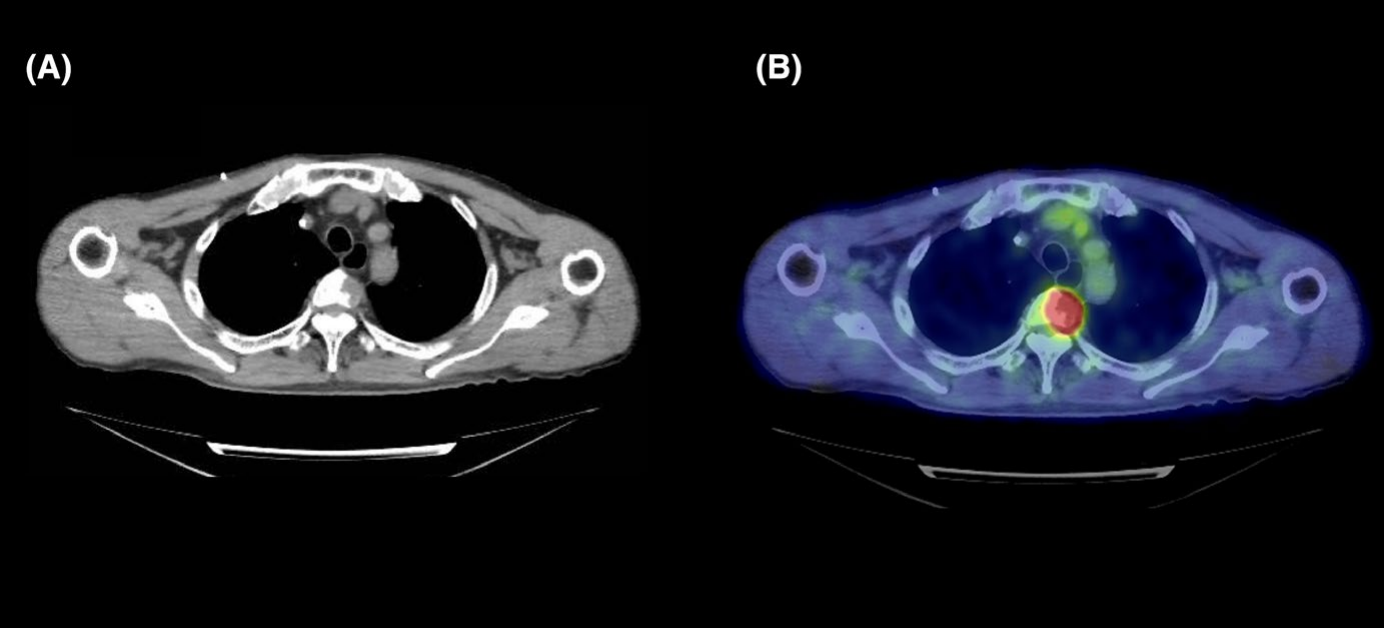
Chest plain computed tomography (CT) scan showing an osteolytic lesion on the left side of the third thoracic vertebra (A). ^18^Fluorodeoxyglucose (FDG)‐positron emission tomography (PET) scan showing high accumulation of FDG at the lesion, which was diagnosed as bone metastasis (B)
